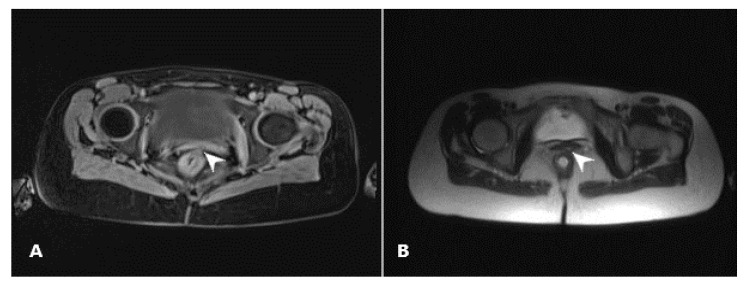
(A) MRI of the pelvis—axial fat-suppressed T1-weighted image with an arrowhead pointing to an abnormal fistulous connection between the rectum and the vagina; (B) MRI of the pelvis—axial T2 weighted image after treatment with an arrowhead pointing to no abnormal fistulous connection between the rectum and the vagina.
